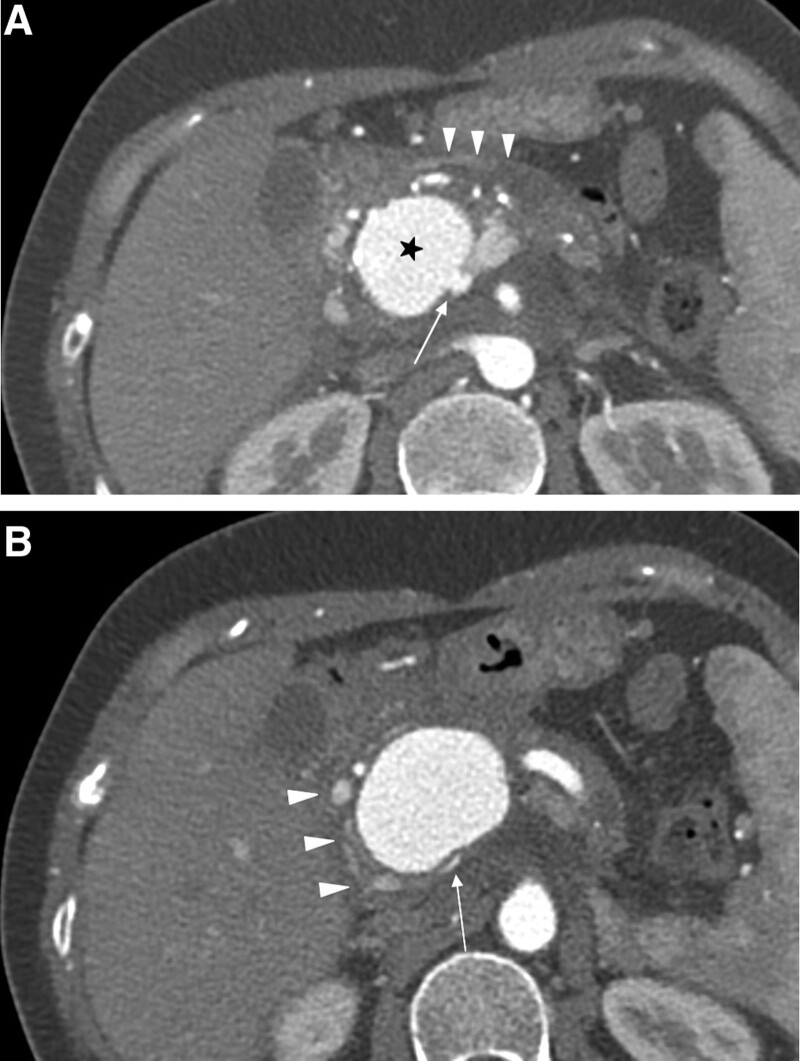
(A) Arterial phase contrast-enhanced CT scan showing GDA pseudoaneurysm (asterisk) communicating with PV (arrow). Pancreatic body shows chunky calcifications and dilatation of the main pancreatic duct (arrowheads). (B) CT scan showing PV stenosis (arrow) caused by large pseudoaneurysm, and underlying chronic pancreatitis. There is developed collateral veins (arrowheads) around GDA pseudoaneurysm. CT = computed tomography, GDA = gastroduodenal artery, PV = portal vein.
